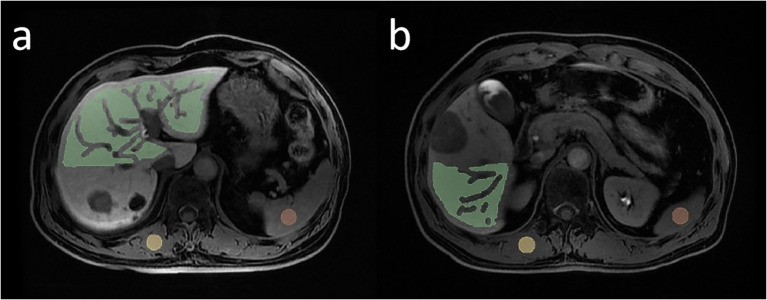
MRI images of HBP phase in two HCC patients. Hepatic regions of ROIs (green) were manually drawn on the maximum cross-sectional plane of the remnant liver after hepatectomy, as well as on two adjacent planes centered on this reference plane. All hepatic ROIs were placed 4–5 mm away from the liver edge to exclude marginal tissue, with strict avoidance of visually identifiable main trunks and primary branches of large hepatic vessels, dilated bile ducts, tubular structures, and focal lesions. Splenic (red) and paraspinal muscle (yellow) reference ROIs were outlined at the corresponding anatomical levels, with a standardized size of 2–5 mm² for all reference ROIs. Quantitative parameters including RLE, CUI, HUI, and LSI were calculated based on the averaged values of all hepatic ROIs. (a) A 66-year-old HCC patient without PHLF, with RLE = 4.90, CUI = 5.84, HUI = 4005.42, and LSI = 3.39. (b) A 50-year-old HCC patient with PHLF, with RLE = 0.35, CUI = 0.55, HUI = 1089.94, and LSI = 0.81.
